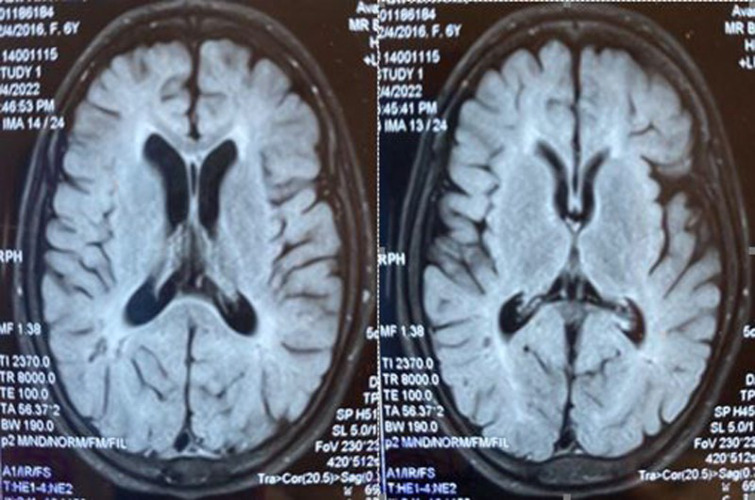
Female sibling axial T1 weighted brain MRI images show periventricular gliosis
